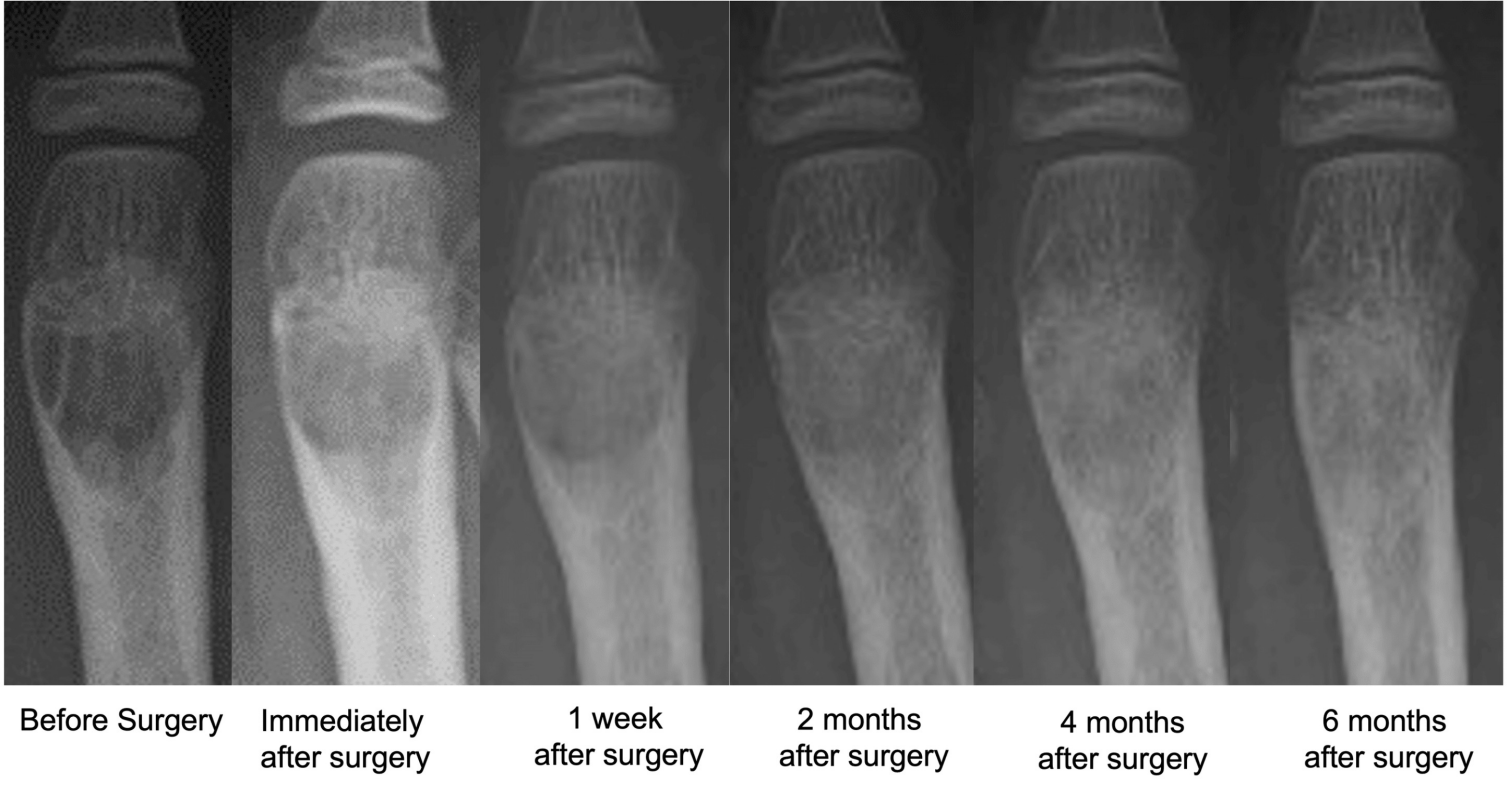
Serial radiographs of a patient with a giant cell tumor of bone Serial radiographs of a 12-year-old boy with a giant cell tumor of bone in the right second metatarsal.
